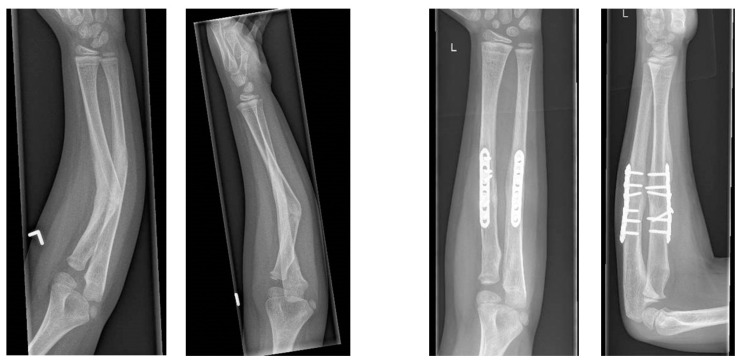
Example of pre- and post-operative radiographs.
